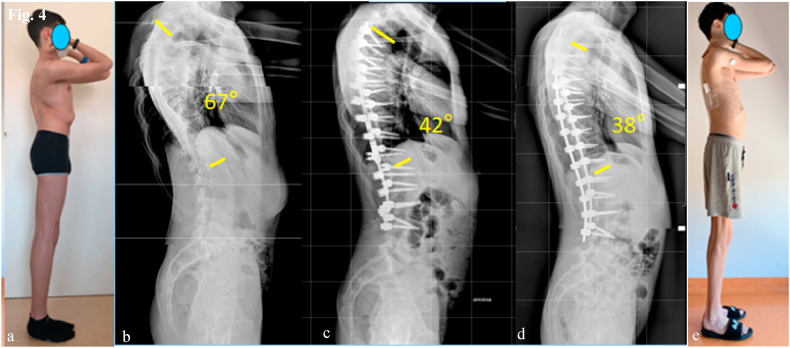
Case 1. Lateral images show: (a) preoperative clinical assessment, (b) preoperative radiograph, (c) correction with MCGR lengthening, (d) final radiographic correction with definitive rods, (e) final clinical correction.
